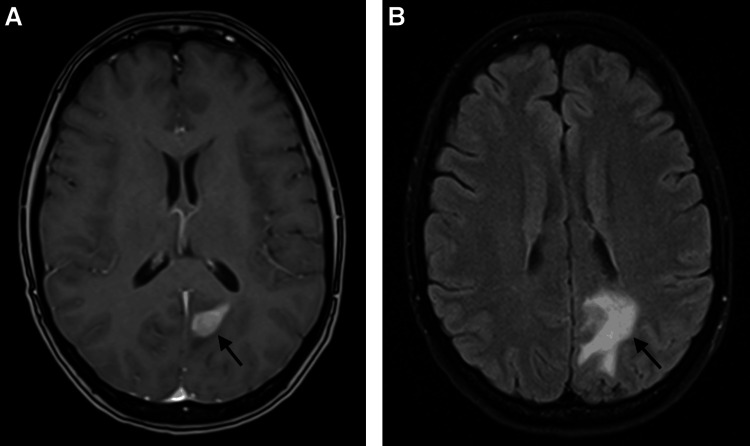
Pre-biopsy and pre-treatment T1 with contrast (A) and T2-FLAIR (B) axial MRI. Pre-biopsy and pre-treatment images demonstrating the left parieto-occipital solitary intracranial plasmacytoma with homogeneous contrast enhancement (A; arrow indicates solitary intracranial plasmacytoma). Surrounding T2 FLAIR hyperintensity is suggestive of vasogenic edema (B; arrow indicates vasogenic edema).
